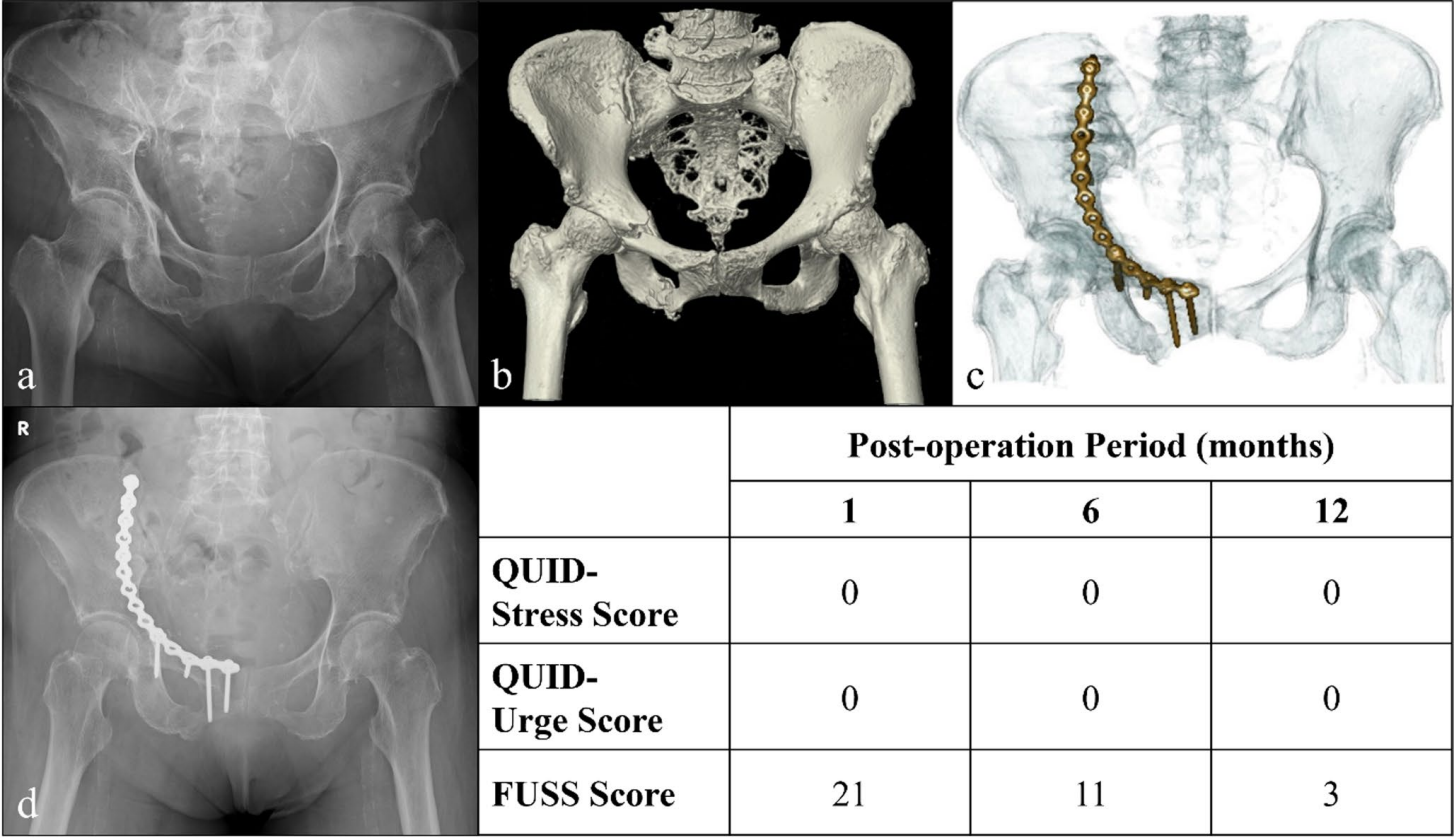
Case presentation of an 84-year-old woman with a PRI classified as LC II (AO B2.2) resulting from a low-energy fall from standing height with related urinary sequelae assessments. (a) and (b) Preoperative X-ray and CT images. (c) Postoperative CT image. (d) Follow-up image at 1 year postoperatively
